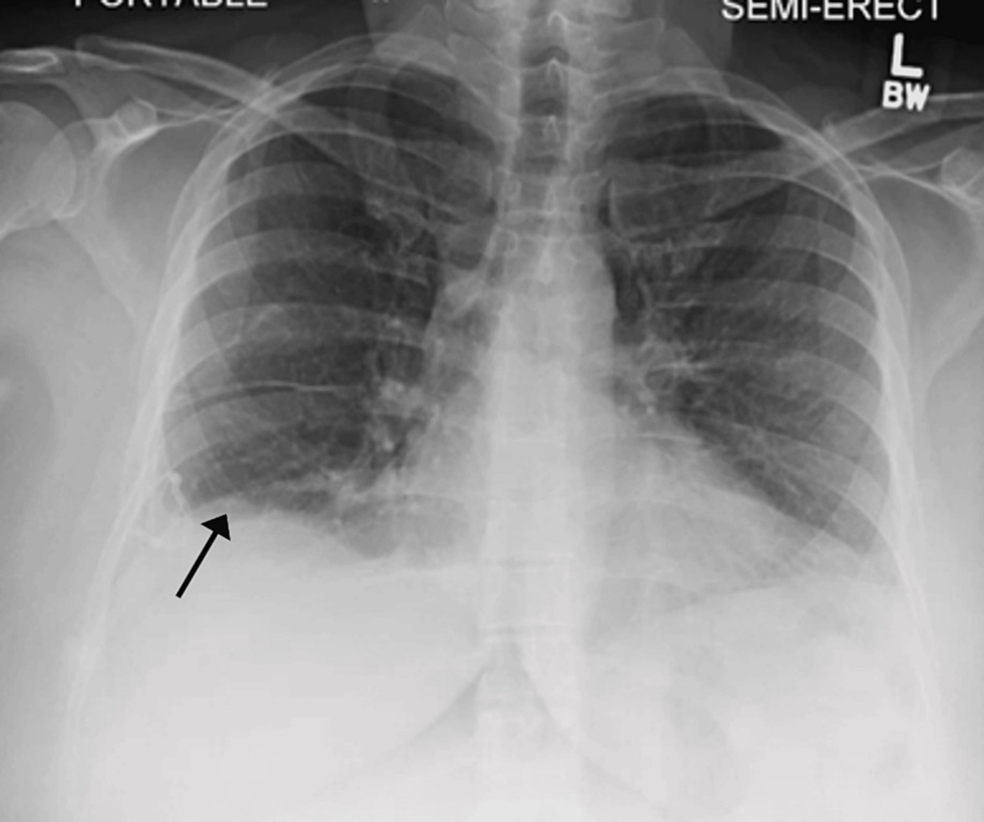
Chest X-ray post thoracentesis, showing resolving effusion. A pigtail catheter was seen in the right lower lung field
